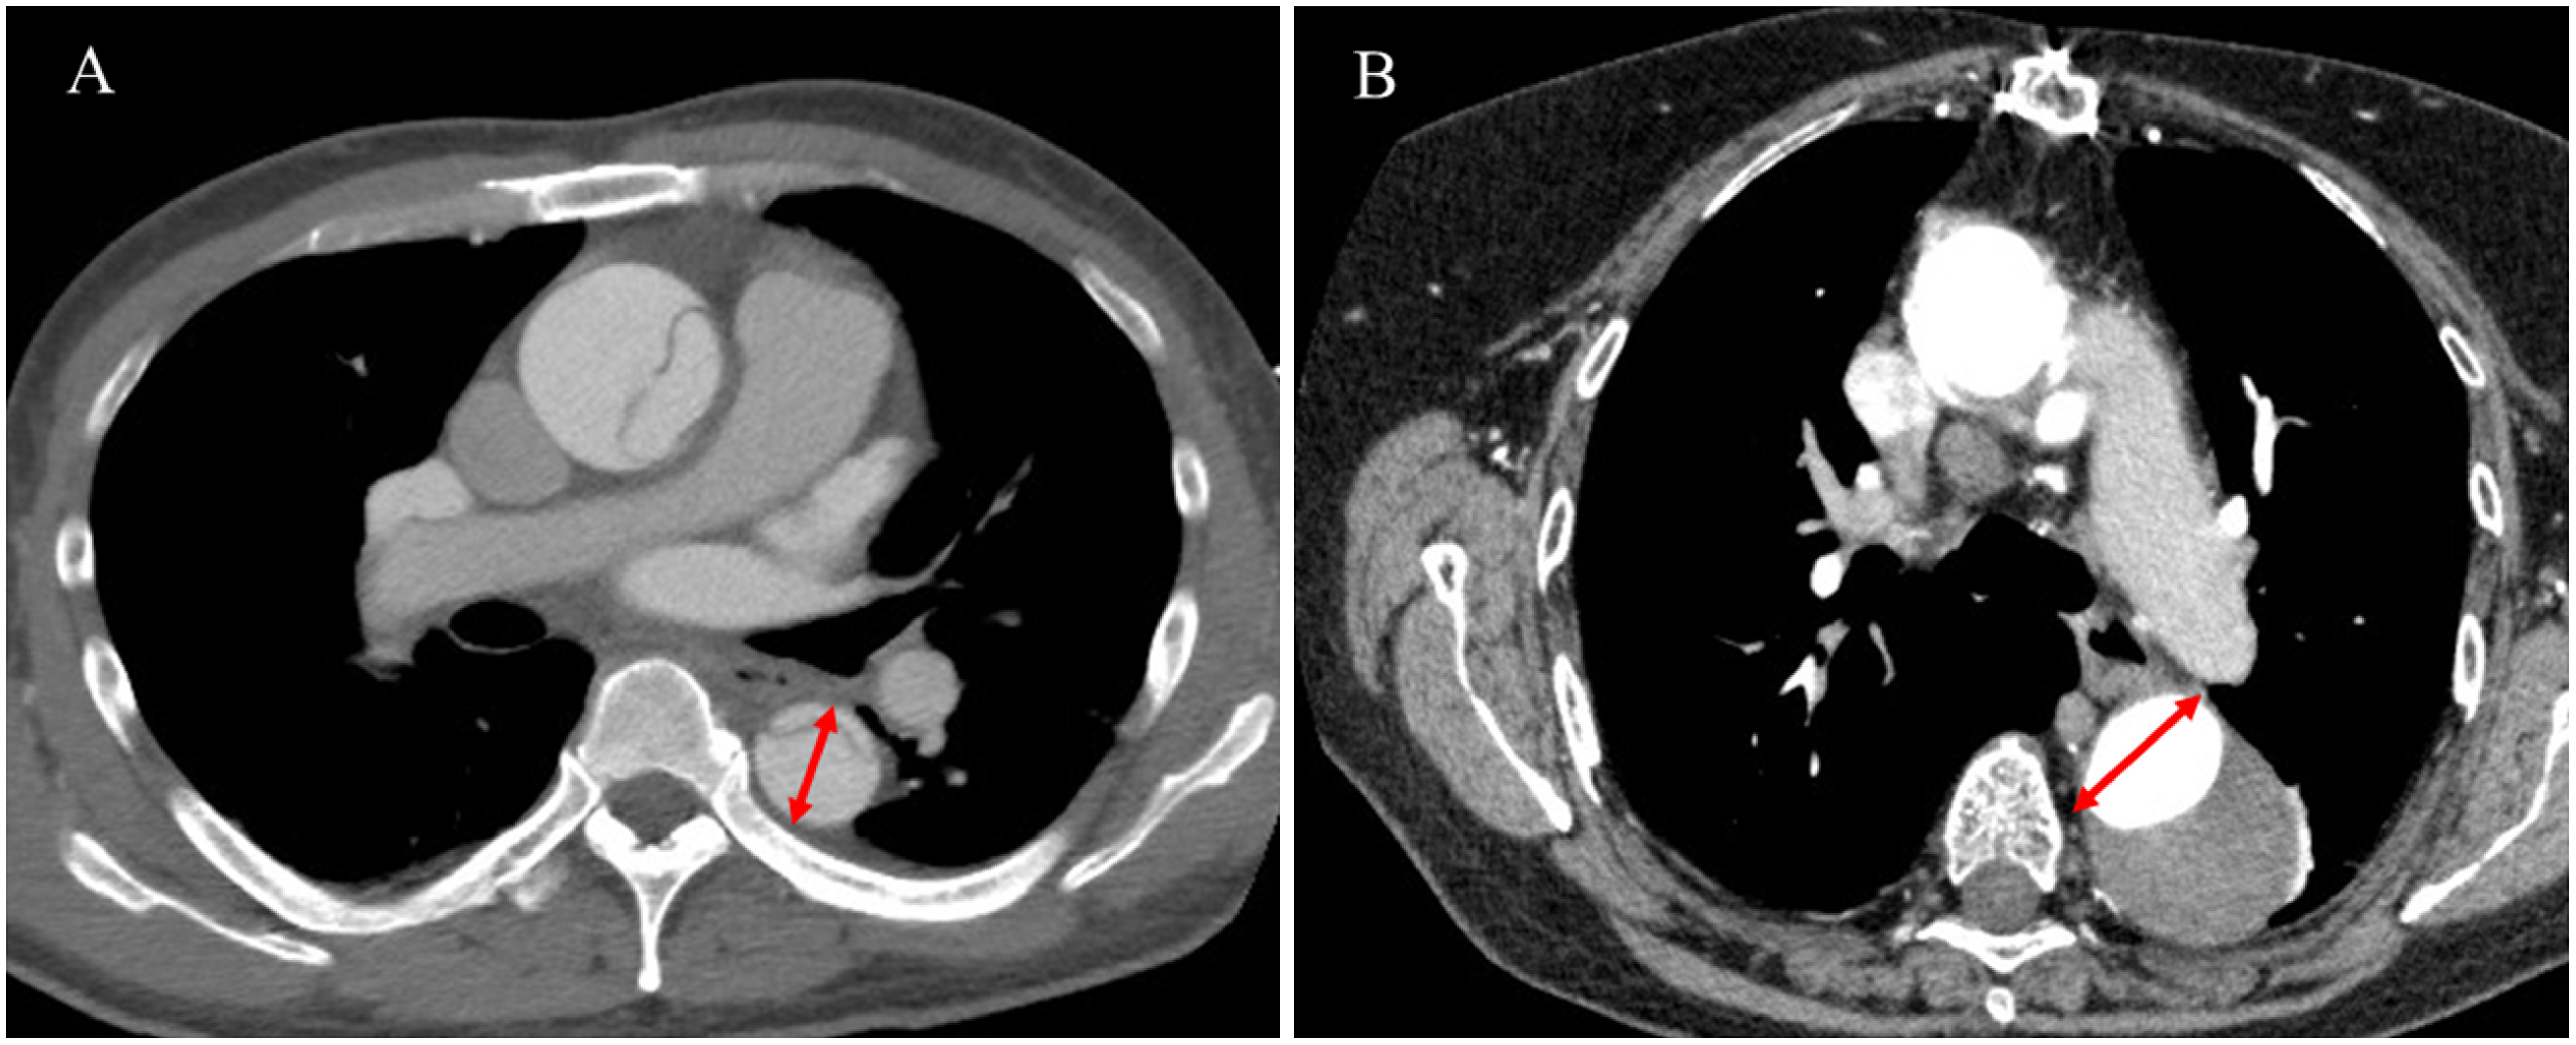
Fig. 1 Preoperative computed tomography images. (A) Acute aortic dissection: The whole aortic diameter of the planned frozen elephant trunk (FET) implantation site is measured (double-headed arrow). (B) Chronic dissection (postoperative residual dissection): The true lumen long-axis diameter of the FET site was measured (double-headed arrow).
